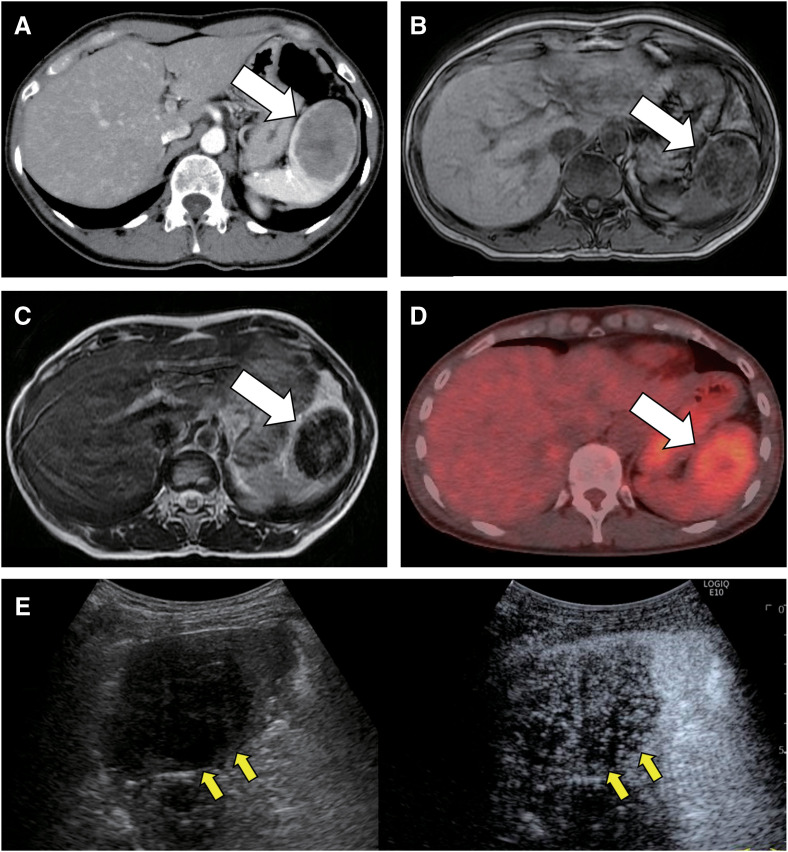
Imaging findings of Case 2. (A) A 60-mm large round tumor with clear borders and heterogeneous internal contrast because of central scarring is enhanced on contrast-enhanced CT (white arrow). (B and C) The tumor is detected with low internal heterogeneity on T1- and T2-weighted MRI (white arrow). (D) PET/CT showing moderate uptake (white arrow). (E) Sonazoid-enhanced ultrasonography shows a mass with a hypoechoic area. The tumor shows delayed and weak enhancement, with areas showing no heterogeneous enhancement (yellow arrow). Central scarring reflecting fibrous stroma is seen in SANT and hamartoma, but not in hemangiomas. MRI T2 low-signal and moderate uptake on PET/CT are observed in SANT. CT, computed tomography; MRI, magnetic resonance imaging; PET/CT, positron emission tomography/CT; SANT, sclerosing angiomatoid nodular transformation
